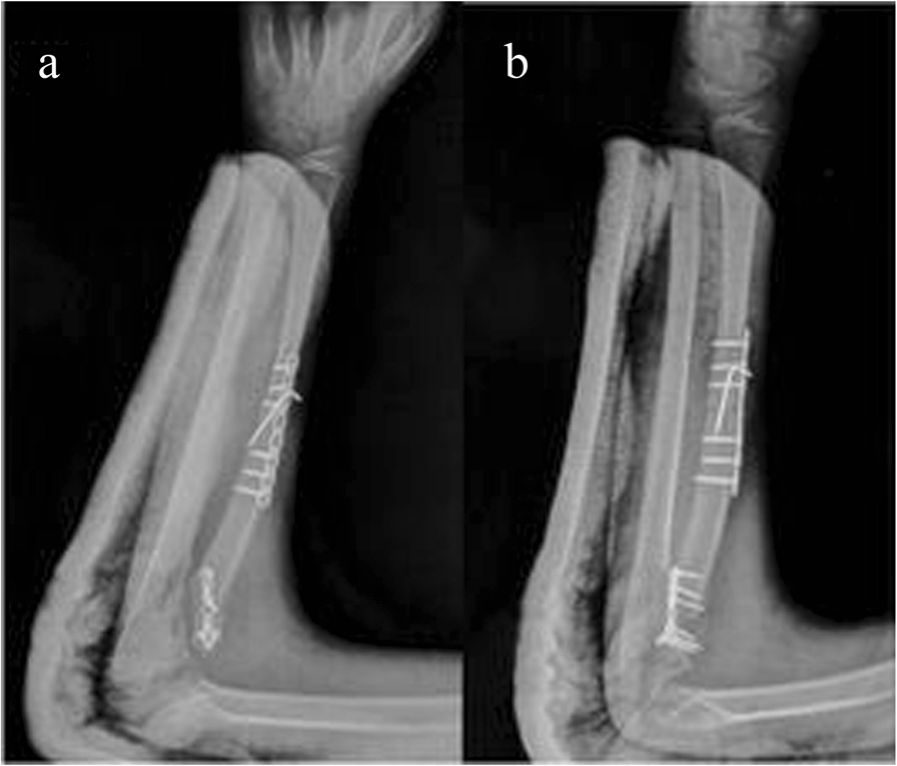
Postoperative radiographs. (a) Oblique and (b) lateral postoperative radiographs showing the resection position, the inner fixation, deformity correction, and restored anatomical relationship of the humeroradial joint.
